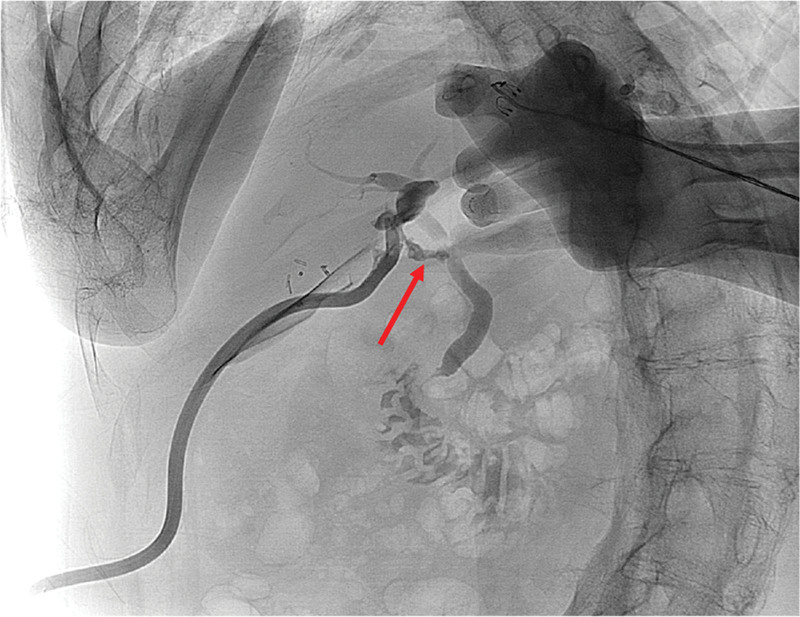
Radiographic view of the surgical drain shows bile leakage at the common bile duct.
